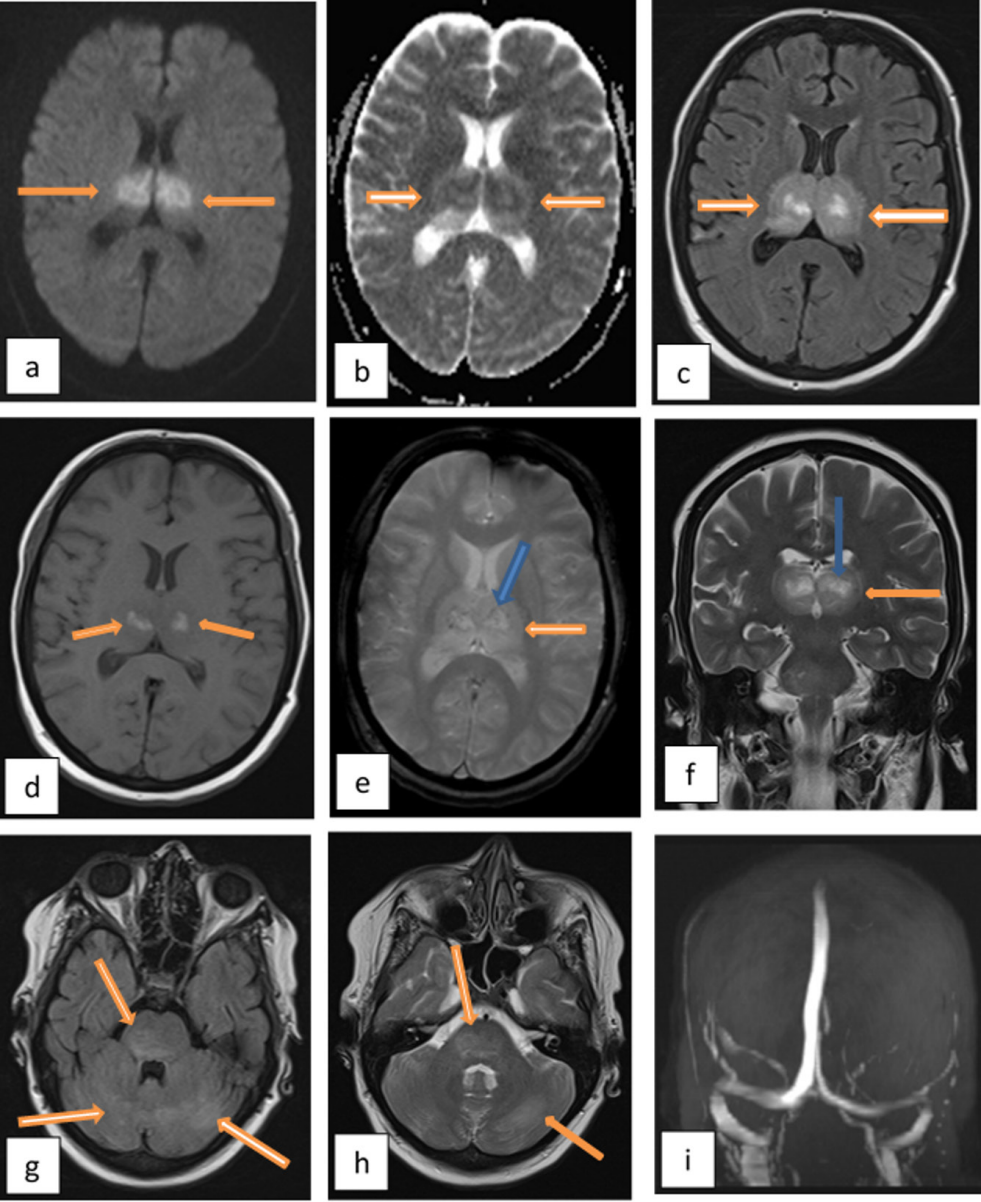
MRI brain on day 4 of admission. (a) Axial DWI and (b) ADC map at the level of thalami show areas of diffusion restriction. (c) axial FLAIR image shows edematous swollen thalami with central necrosis. (d) axial *T*_1_WI shows bright signal at the center of both thalami denoting hemorrhage. (e) axial gradient image at the same level with the mild dark blooming signal at the site of hemorrhage (blue arrow) surrounded by edema (orange arrow). (f) coronal *T*_2_WI shows the edematous thalami (orange arrow) with central necrosis (blue arrow). (g) axial FLAIR and (h) axial *T*_2_WI at the level of the posterior fossa shows the bright signal of edema at the cerebellum and pons. (i) MRV with the patent normal deep cerebral venous system. ADC, apparent diffusion coefficient; DWI, diffusion-weighted imaging; FLAIR, fluid-attenuated inversion-recovery; MRV, MR venography.
